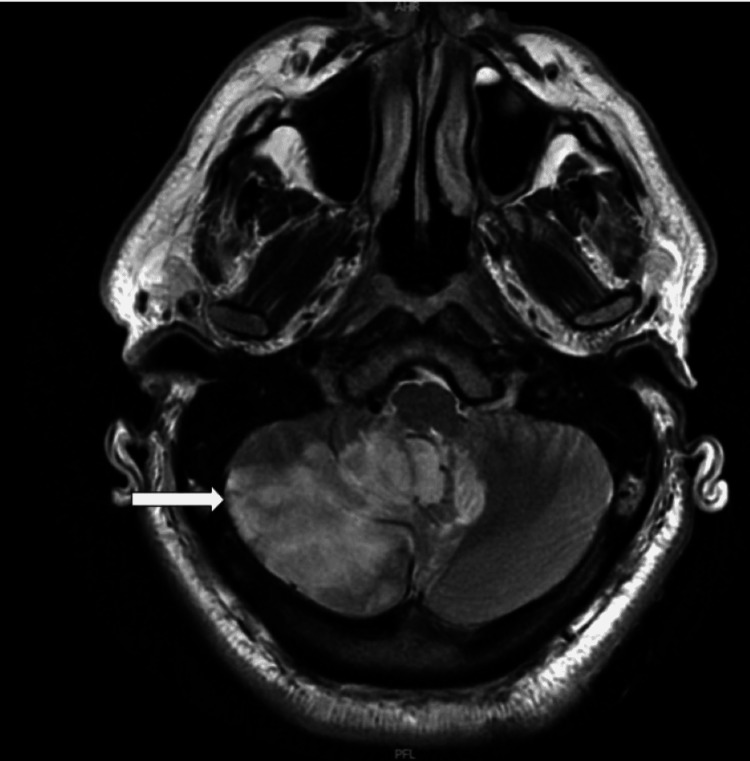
Right sided hyperintense lesion in the cerebellum with left shift in brain magnetic resonance imaging (MRI) Brain magnetic resonance imaging (MRI) performed with axial plane utilizing gradient echo sequence which revealed right sided hyperintense lesion in the cerebellum with minimal midline shift. "
